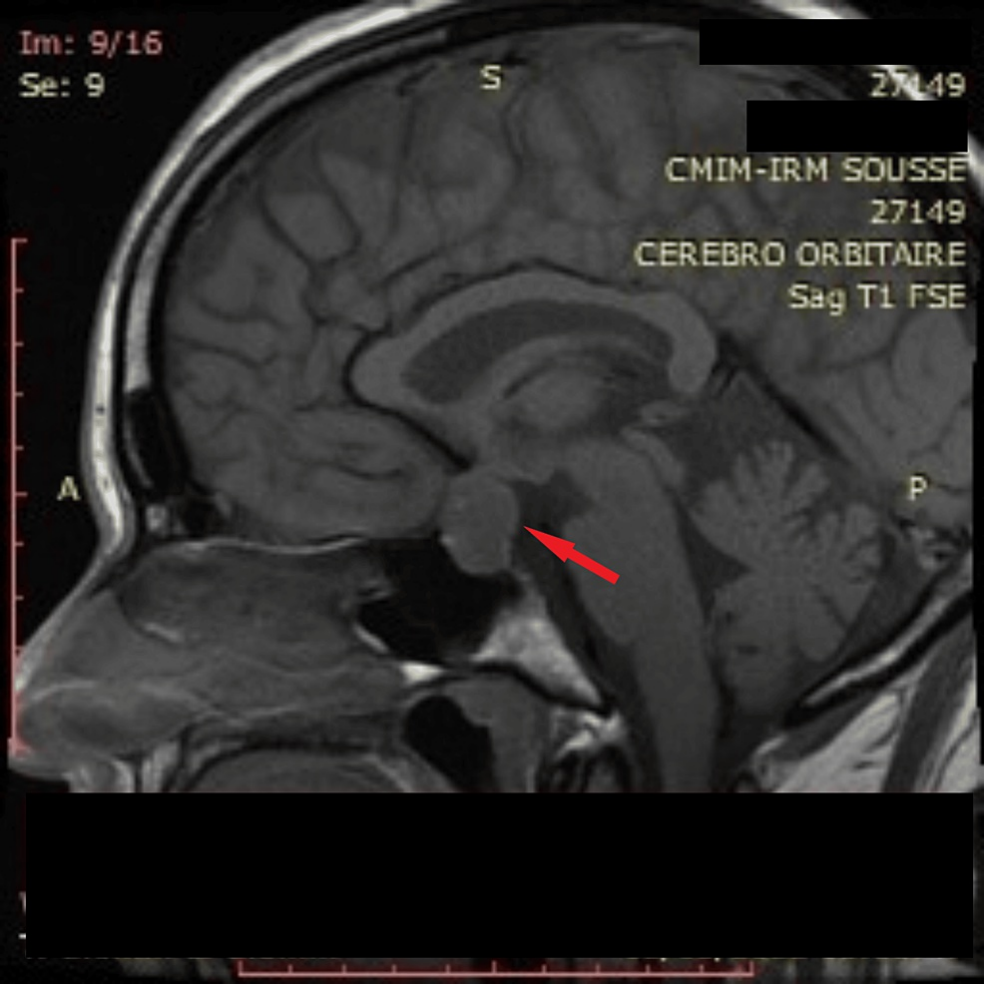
Preoperative hypothalamic-pituitary MRI sagittal section T1-weighted sagittal section of a 17 x 18 x 19 mm pituitary adenomatous lesion (red arrow).
